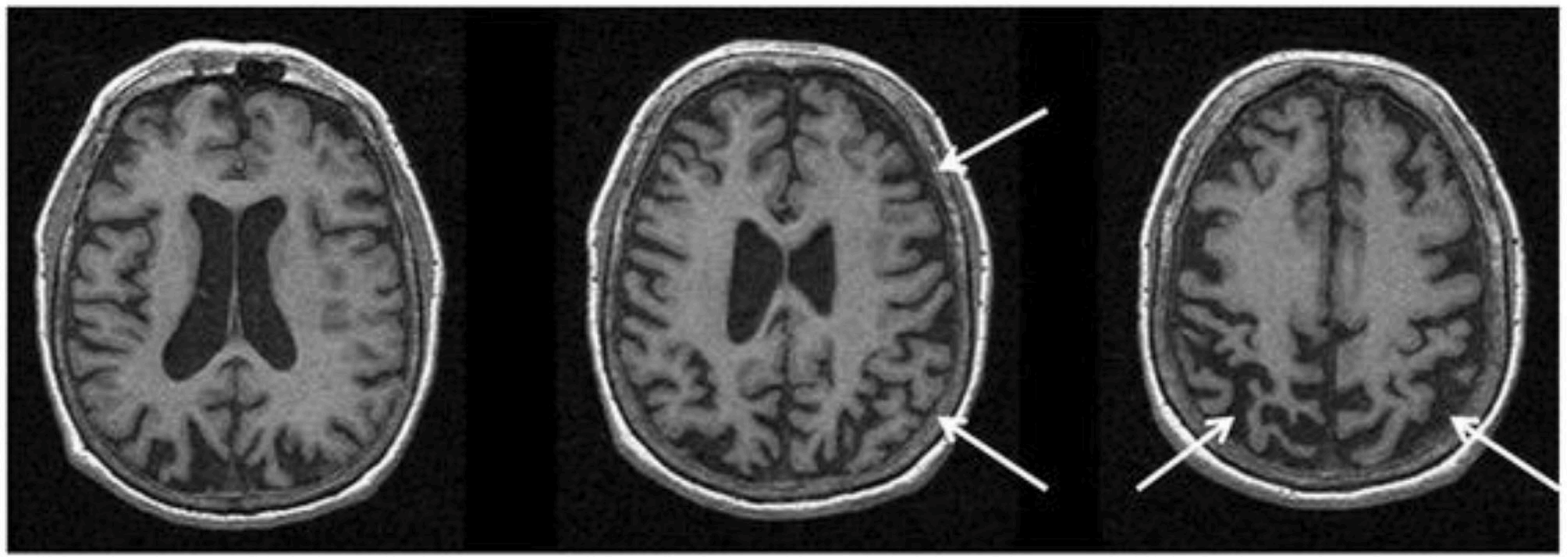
Magnetic resonance imaging of a patient with a pathology-proven diagnosis of corticobasal degeneration. Serial axial T1-weighted sequences are presented showing right greater than left parietofrontal atrophy commonly seen in corticobasal syndrome. Figure reproduced from Saeed et al. (10), under the Creative Commons Attribution License 4.0 (https://creativecommons.org/licenses/by/4.0/).
